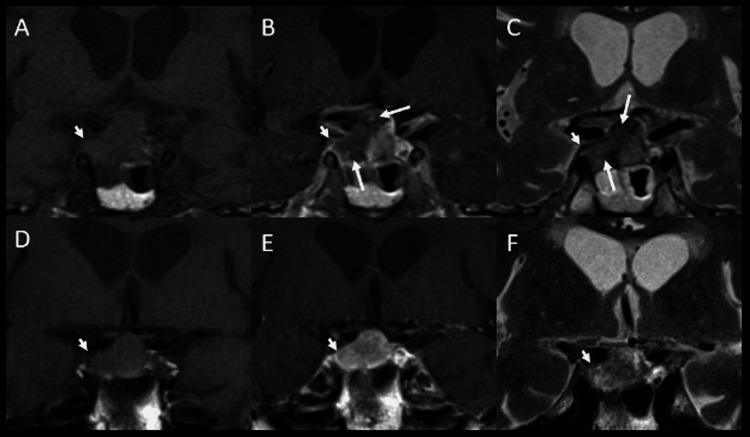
MRI sella with pre-contrast T1 (A,D), postcontrast T1 (B,E), and T2 (C,F) weighted imaging with comparison MRI from four years earlier (D,E,F) demonstrate pituitary macroadenoma with suprasellar and right cavernous sinus extension (arrowheads). There is development of heterogenous enhancement with a large non-enhancing component corresponding to interval necrosis and hemorrhage within the adenoma compatible with pituitary apoplexy (arrows in B). Changes in T2 signal with hypointensity (arrows in C) is due to evolving blood products.
